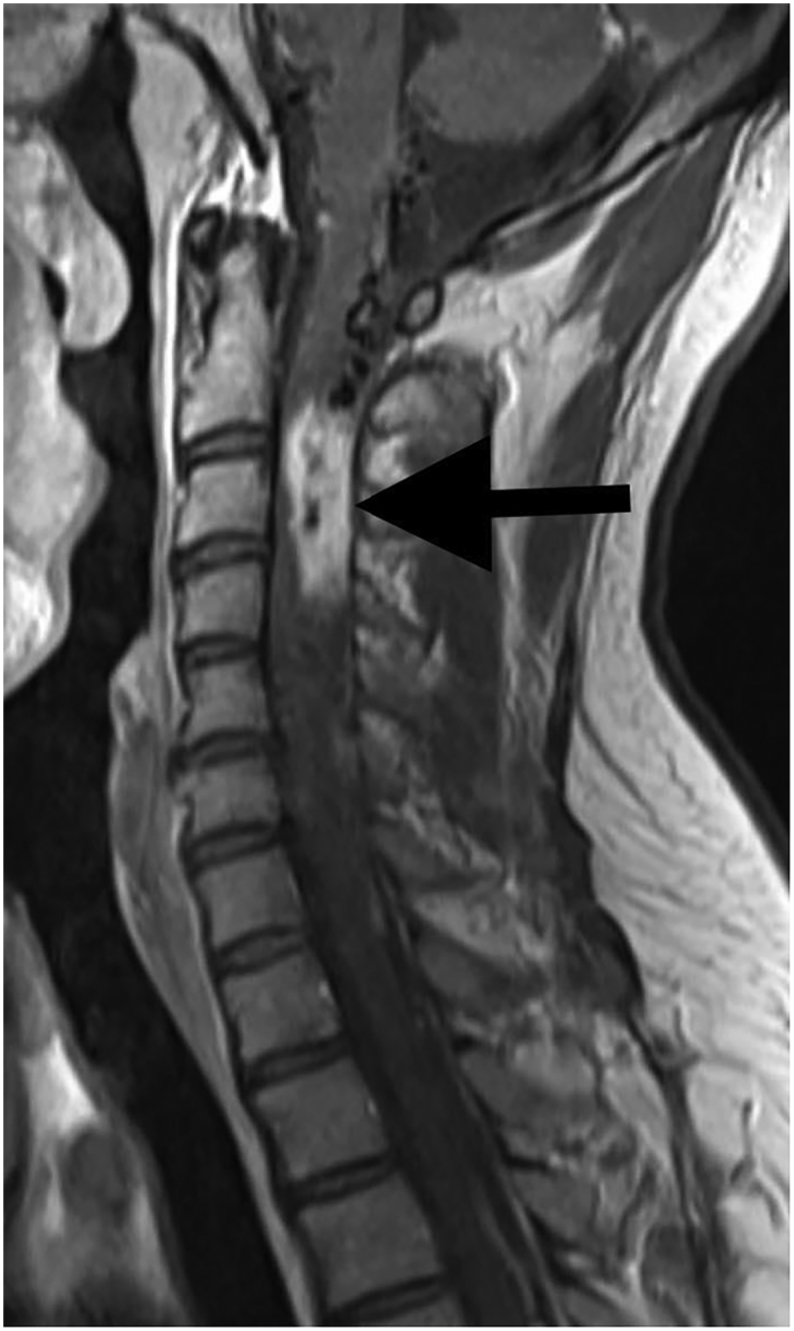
T1-weighted postcontrast MRI showing spinal hemangioblastoma at C2/C3/C4 cervical vertebrae level (arrow).
